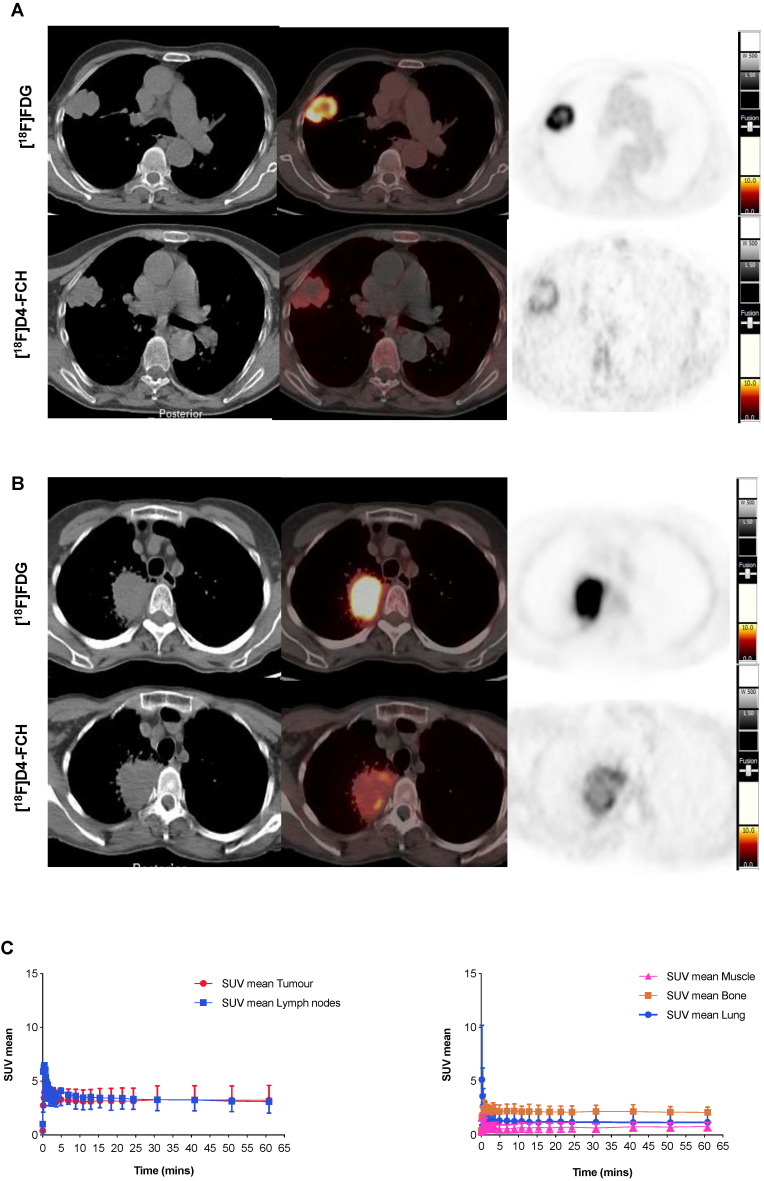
[^18^F]D4-FCH and [^18^F]FDG Tissue uptake. (A) Axial views on CT, fused PET/CT and PET for diagnostic [^18^F]FDG and [^18^F]D4-FCH in a patient with squamous cell carcinoma of the right lung (patient 3 - see Table 2); (B) similar images in a patient with an adenocarcinoma of the right lung (patient 13 - see Table 2), SUV intensity threshold 0-10; and; (C) Mean decay-corrected time-activity curves (TACs) for normal tissue (normal lung, bone, including bone marrow, and muscle) and tumor and lymph nodes in dynamic PET imaging.
